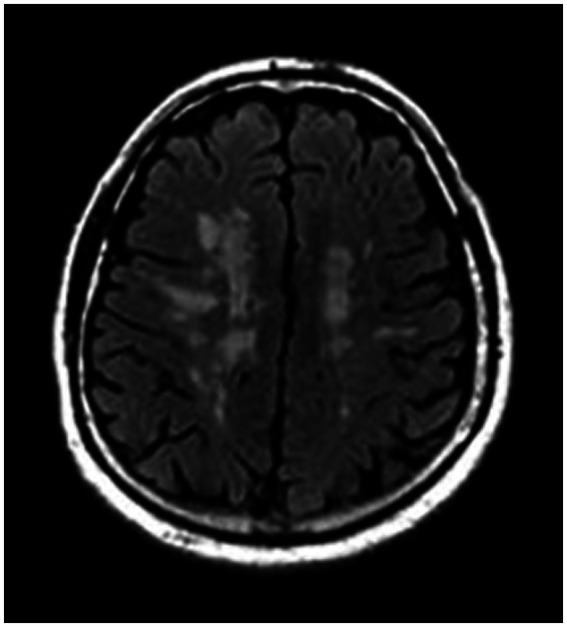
White matter lesion in a patient with chronic migraine headache, FLAIR sequences of MRI.
